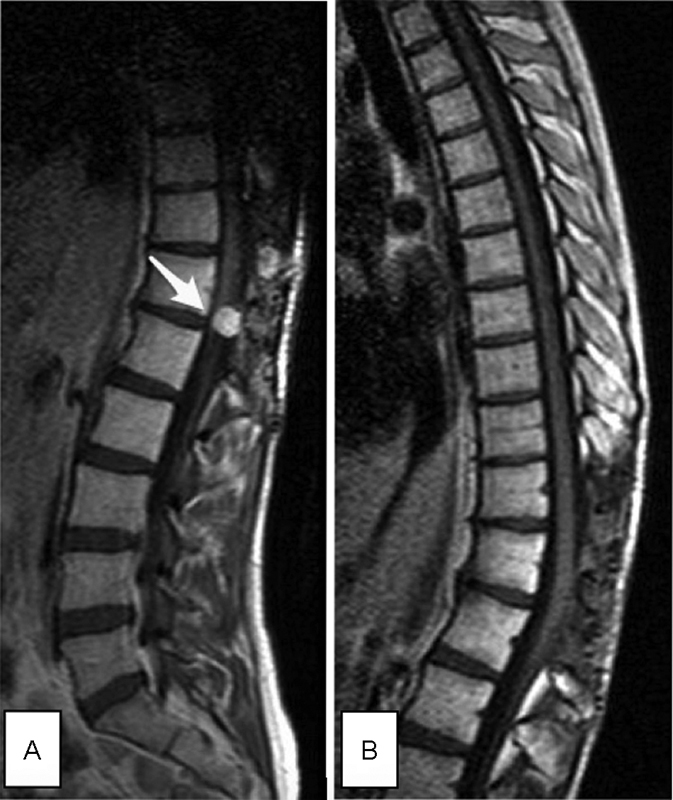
First recurrence of the tumor in 2006 in MR imaging. (
